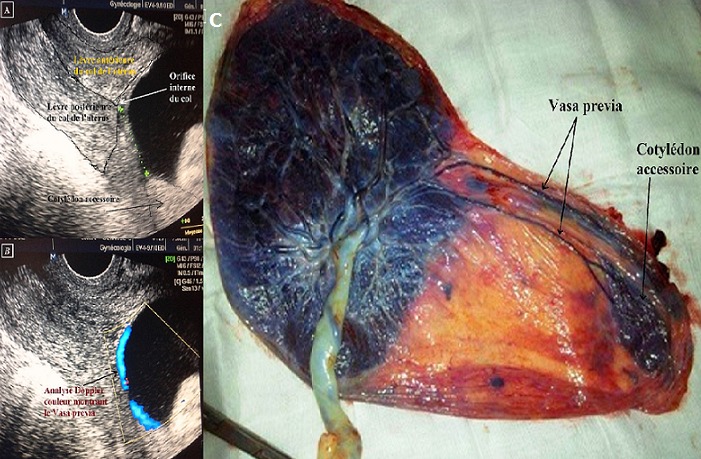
A) échographie endovaginale montrant un lobe accessoire postérieur à 24 mm de l’orifice interne du col; B) analyse Doppler montrant un vaisseau preavia; C) aspect macroscopique montrant le lobe accessoire relié au placenta par de larges vaisseaux
